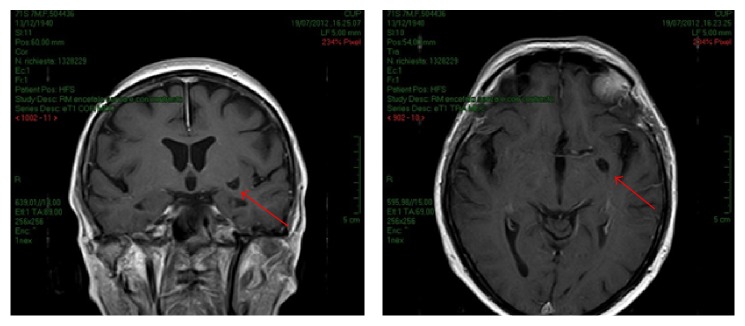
A large CSF perivascular space of the anterior perforated substance, in the basal portion of the left lentiform nucleus evidenced by a second MRI (indicated with arrows).
